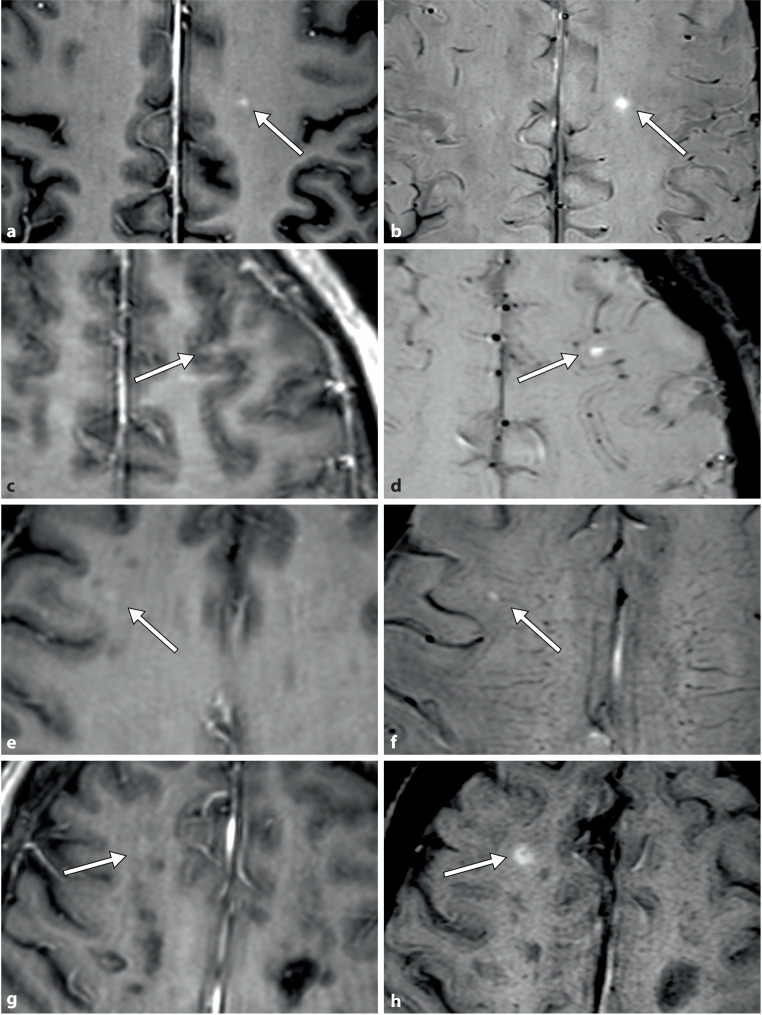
Examples of cases with improved enhancing lesion visualization with post-contrast susceptibility-weighted imaging (SWI+C). Each row corresponds to the same patient and MRI session, centered on the same lesion. The left column corresponds to contrast-enhanced T1-weighted imaging (T1WI+C), while the right column corresponds to SWI+C. The case in the top row was identified on T1WI+C but was more conspicuous and more straightforward to visualize on SWI+C. The remaining cases were initially missed on T1WI+C by one or both readers, but some subtle enhancements on T1WI+C became visible after identifying them on SWI+C
